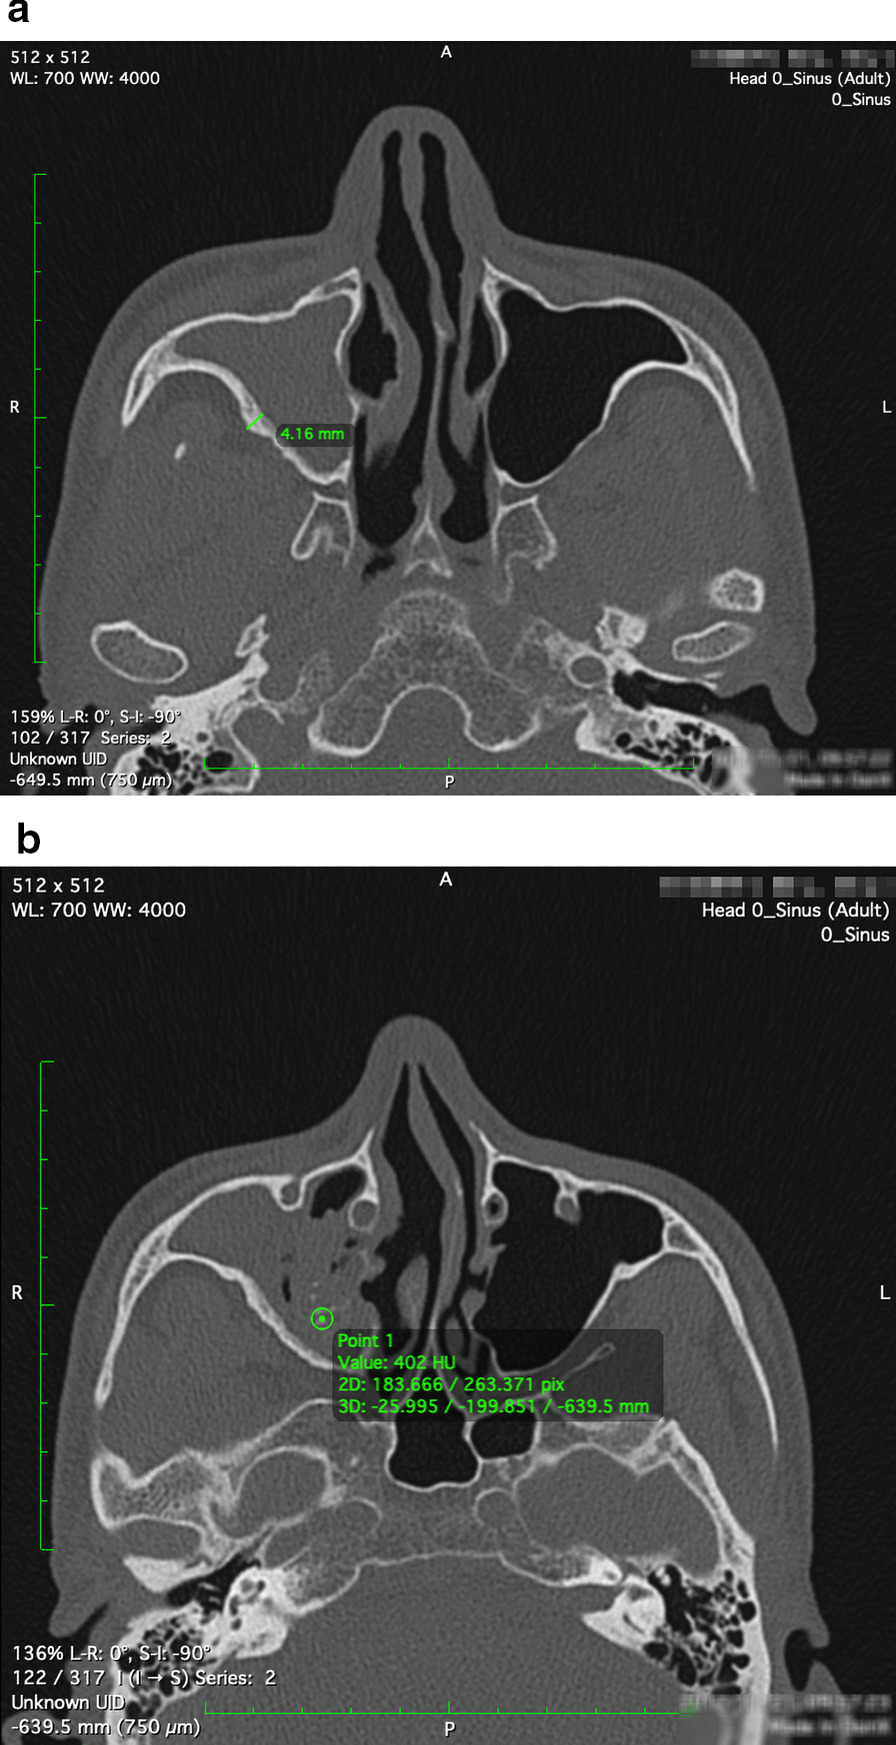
Sinus CT measurements were performed by OsiriX® software. a The thickness of the posterior lateral wall of the maxillary sinus; b the highest CT value of the high-density area in the maxillary sinus
